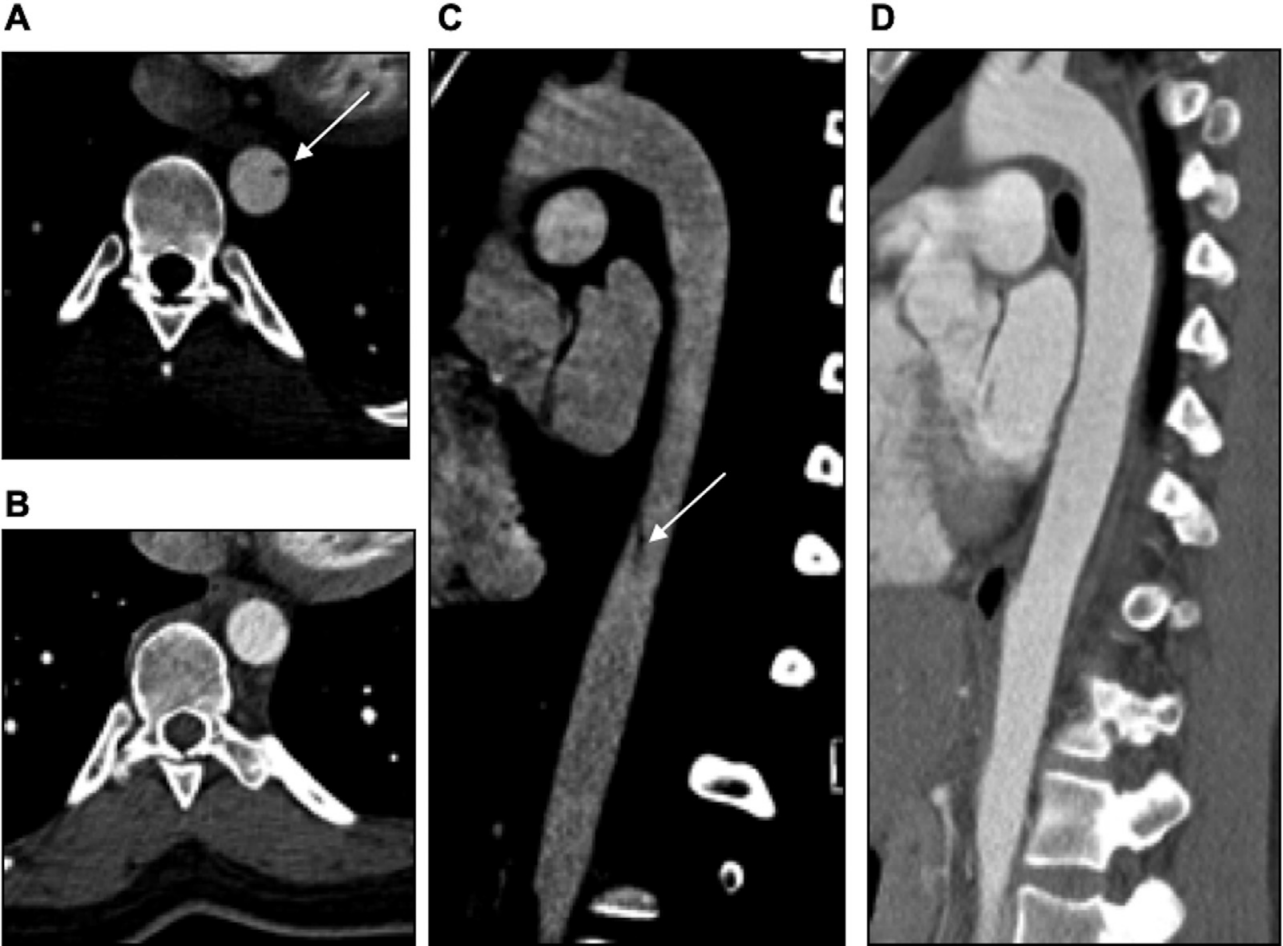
Grade I minimal aortic injury in the descending thoracic aorta on baseline trauma CTA in axial (
